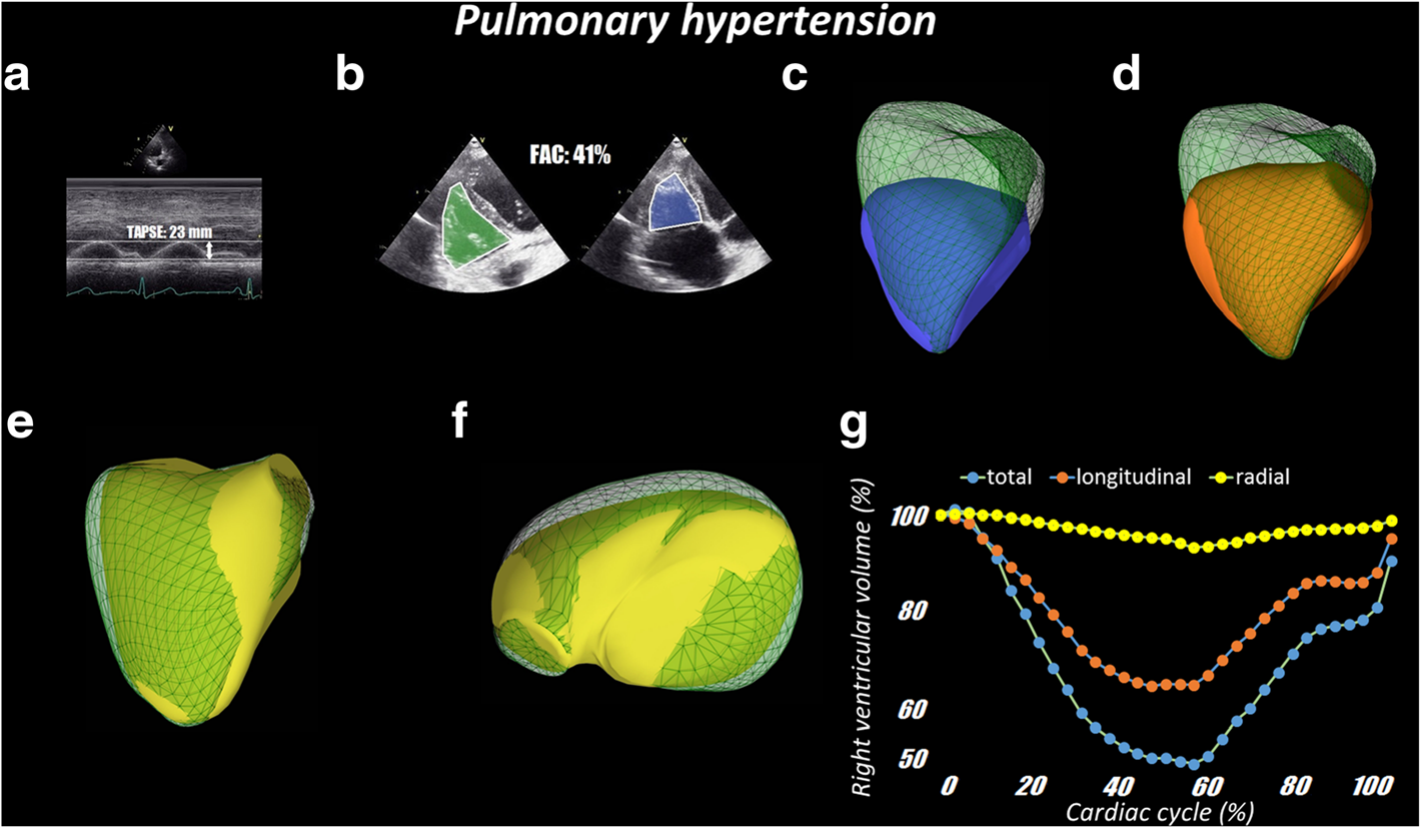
Representative pulmonary hypertension patient. Panel a: TAPSE (23 mm) was normal. Panel b: FAC (41%) was also normal indicating preserved RV systolic function. Panel c: RV end-diastolic (92 ml) and end-systolic (48 ml) volumes as well as RV ejection fraction (48%) obtained from 3D echocardiography data sets showed normal RV systolic function. Panel d: the longitudinal displacement appears supernormal. Panel e and f: By removing the longitudinal and anteroposterior components of RV volume change, the radial displacement of RV wall appears dramatically reduced. Panel g: Quantitative analysis of the relative contribution of longitudinal and radial RV wall displacement to global RV volume change confirms the significant reduction of radial displacement and an increase of longitudinal displacement as the main mechanism to preserve RV stroke volume and ejection fraction in chronic, compensated RV pressure overload pathophysiology (pulmonary artery systolic pressure: 69 mmHg)
